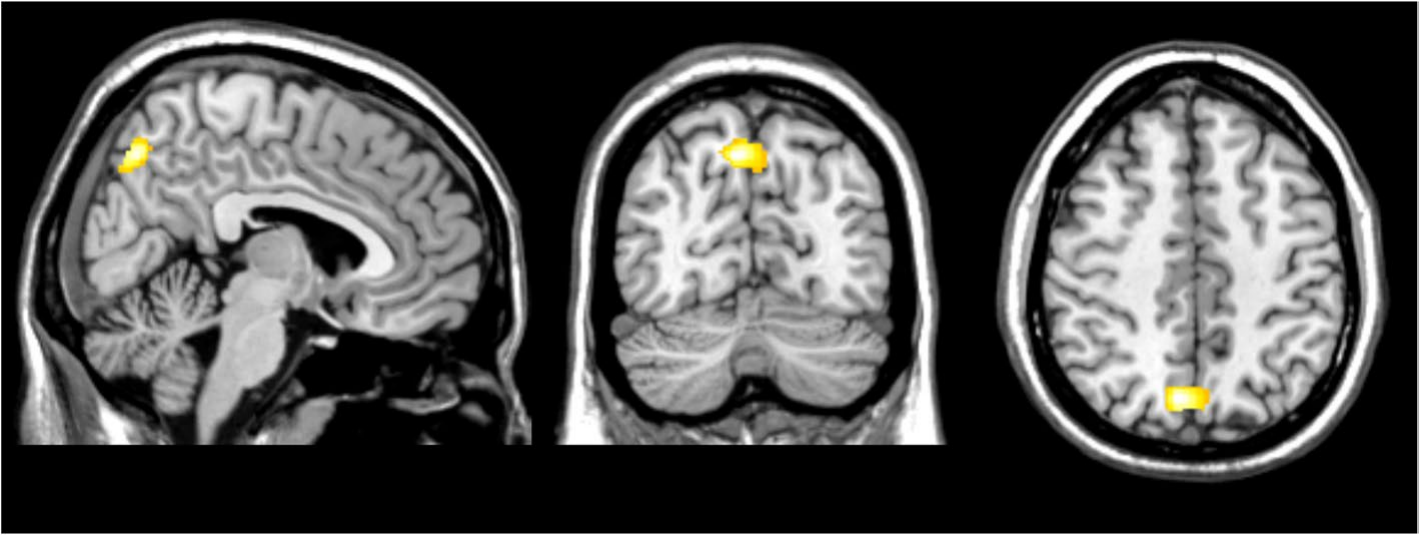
Regions of increased gray matter volume in Cluster One compared to Cluster Two overlaid onto the sagittal (x = 86), coronal (y = 56), and axial (z = 119) planes of a T1-weighted brain MRI template, radiologically oriented. The threshold has been set to *p*=0.001_unc_ for displaying purposes. Additional details can be found in the main text and Table 2
